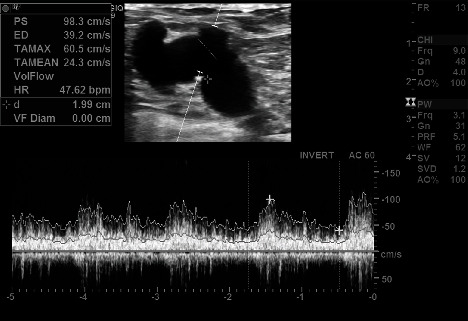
Duplex ultrasound showing an abnormally shaped AV fistula with associated pseudoaneurysm.
